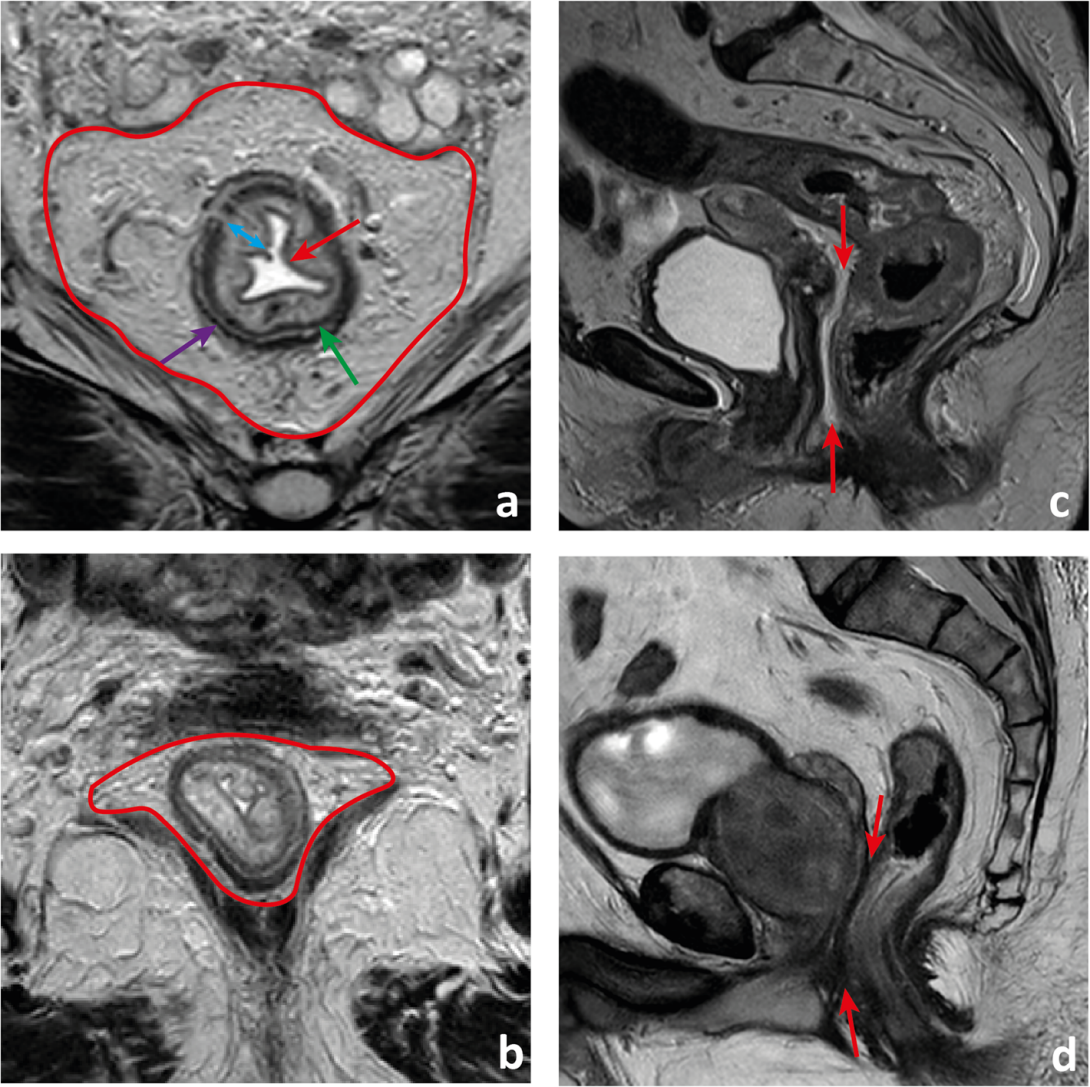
The rectal wall. The mucosa is visible on T2-weighted imaging as a regular thin (≈ 1 mm) hypointense line delimiting the lumen (red arrow in a). The submucosa is hyperintense and of variable thickness (double-headed blue arrow in a). It may not be visible when the rectum is distended. The *muscularis propria* is composed of an inner circular layer (green arrow in a) and an outer longitudinal layer (purple arrow in a) which may be separated by a thin layer of fat, as in the example shown. Lying externally to it is a cushion of mesorectal fat (delimited in red in a) that tappers inferiorly (delimited in red in c) and anteriorly, where it can be thin (between arrows in c) or even invisible (between arrows in d), in which case the mid/low rectum appears juxtaposed to the Dennonvillier fascia
